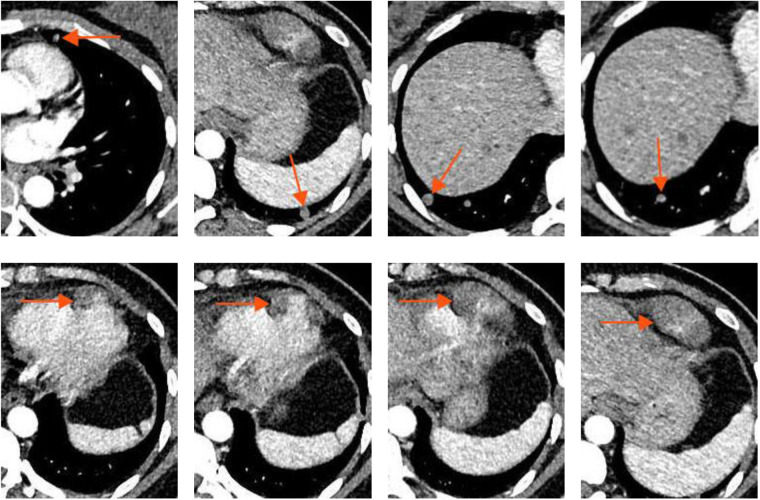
Contrast-enhanced chest CT.
